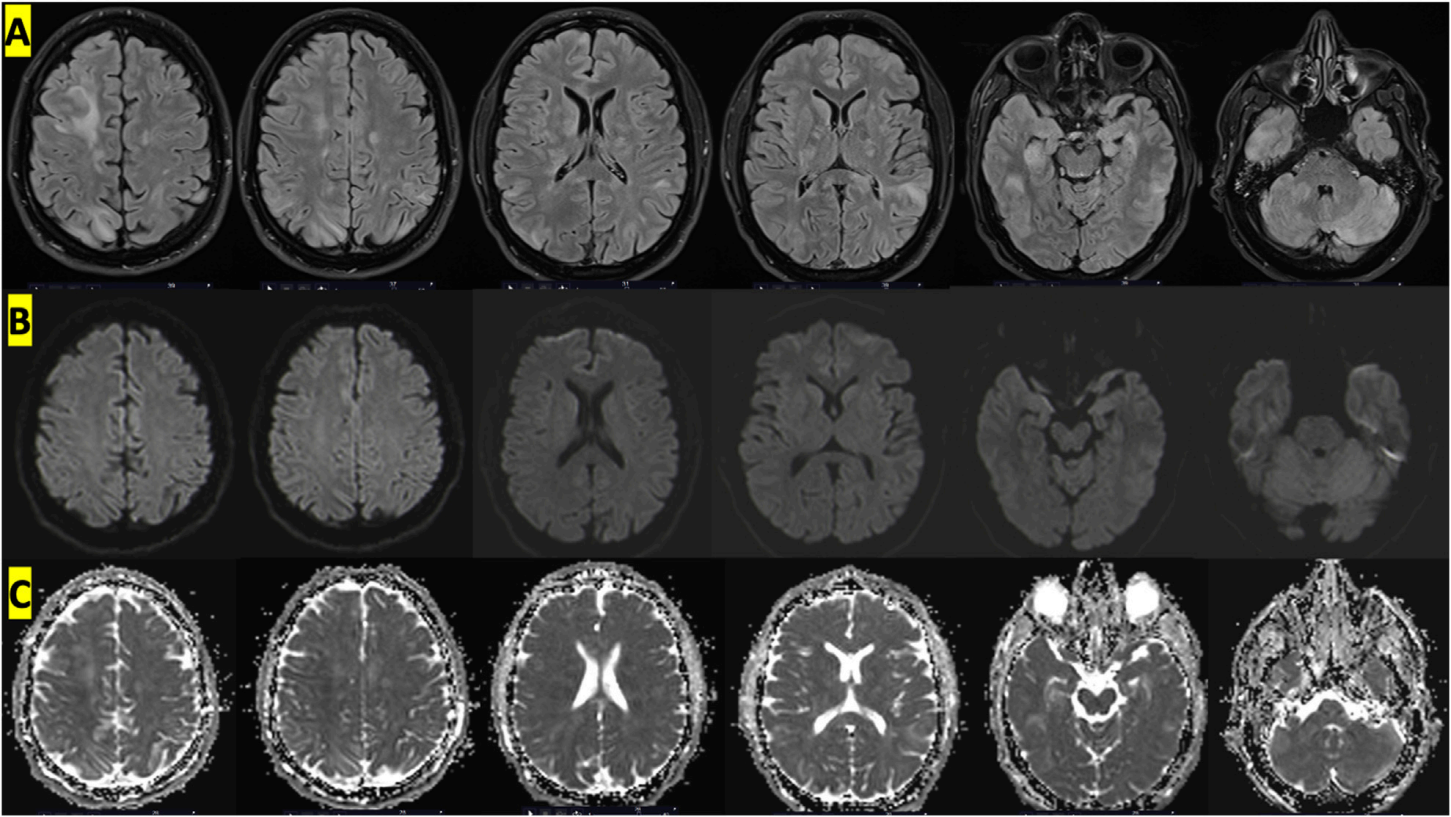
Cerebral MRI with an appearance suggestive of vascular edema showing diffuse cortico-subcortical, supratentorial and subtentorial involvement on FLAIR sequences. (A) T2 FLAIR sequences showing hyperintense signals at different levels, (B) absence of hyperintensities in DWI sequences and (C) increased value on ADC map.
